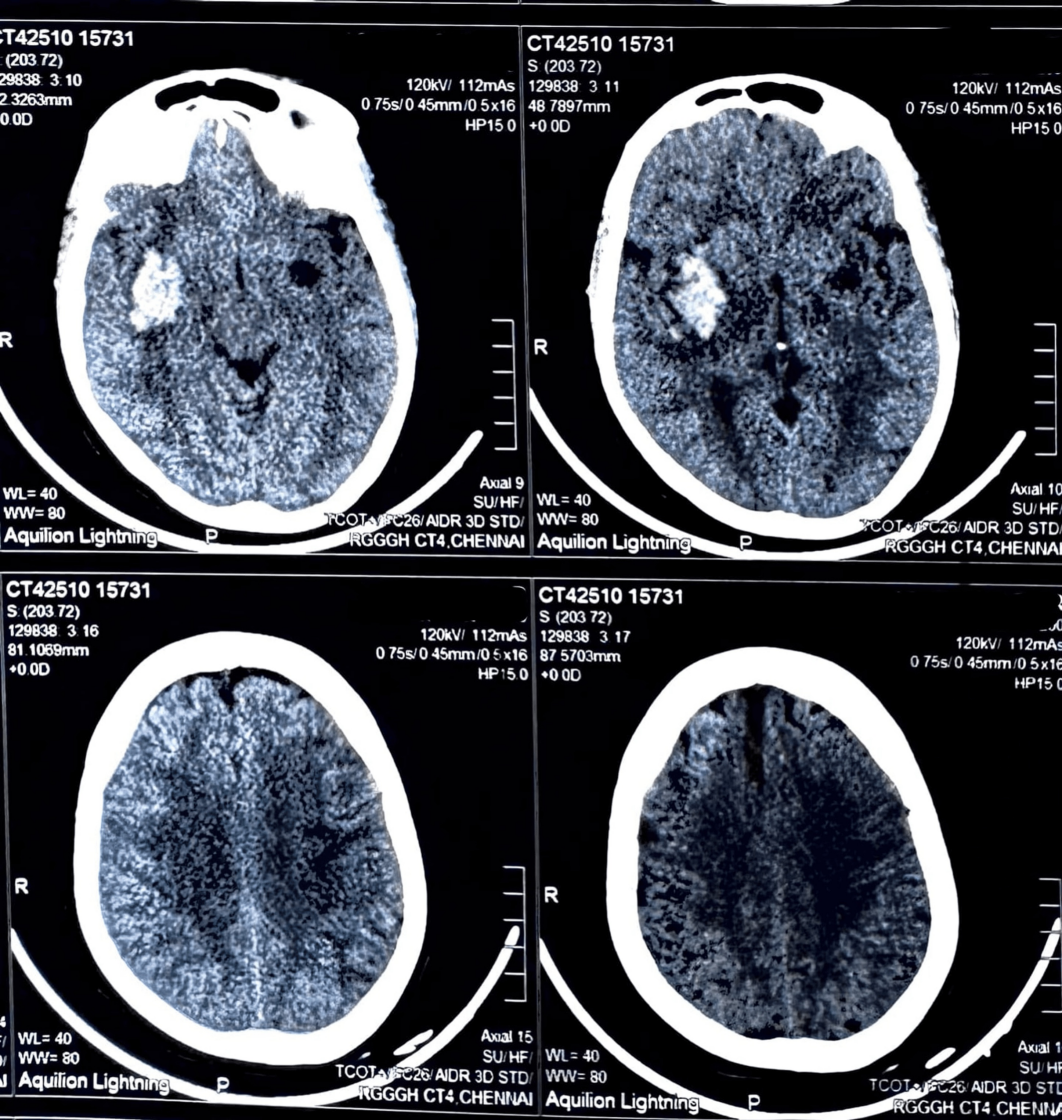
CT brain of the patient at the time of admission
